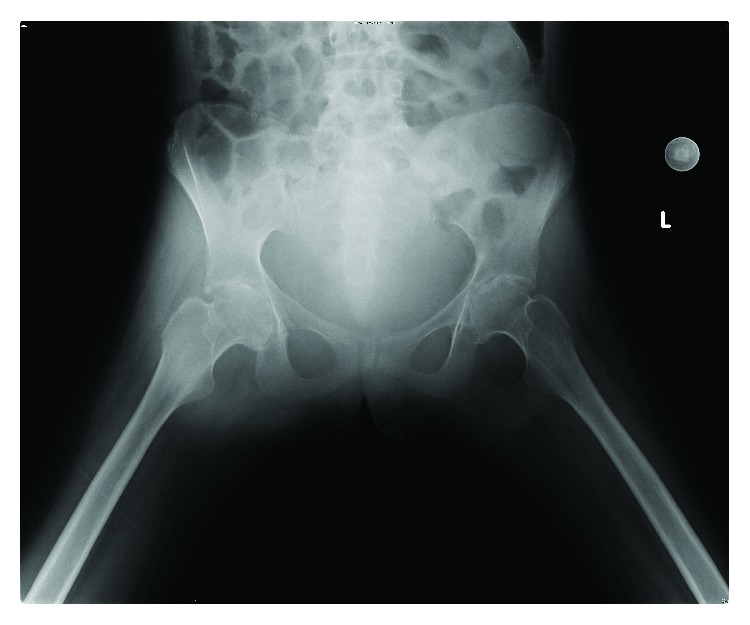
Plain hip radiographs at age 19.
